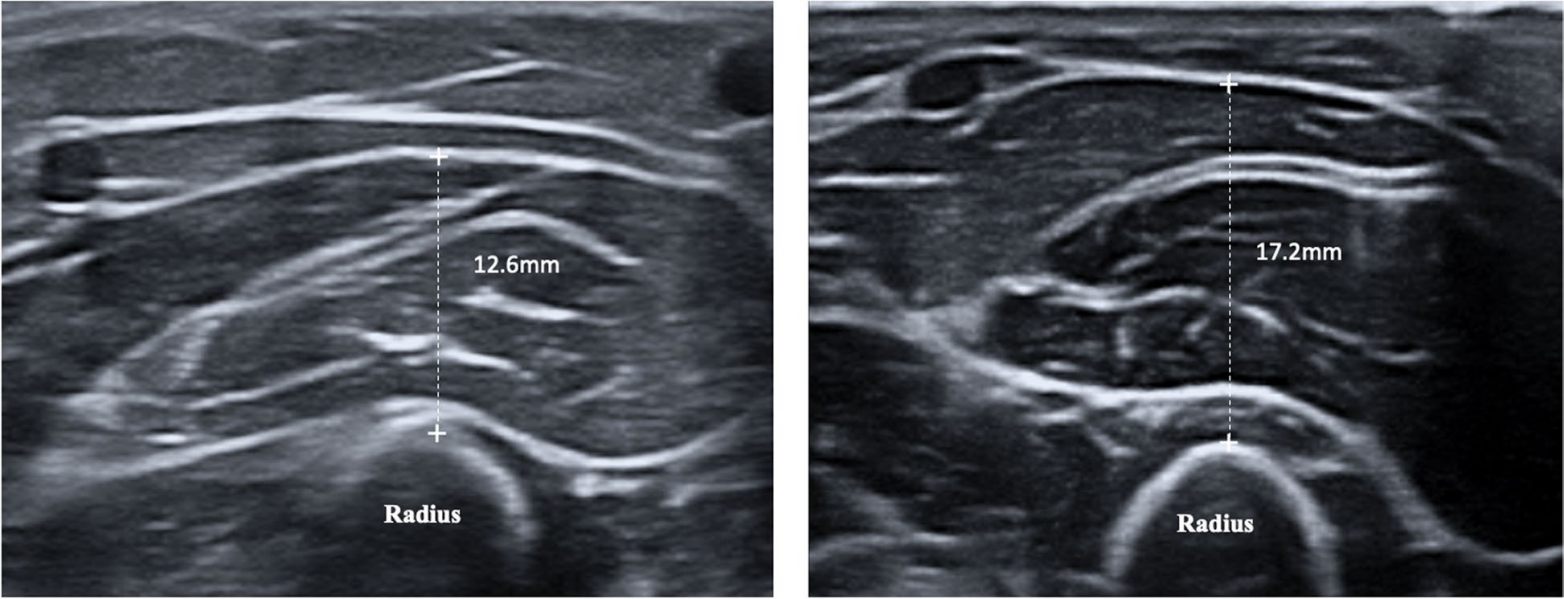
Forearm muscle thickness sonogram, Legend: Image obtained in a patient (left) and healthy volunteer (right), showing quantitative differences
